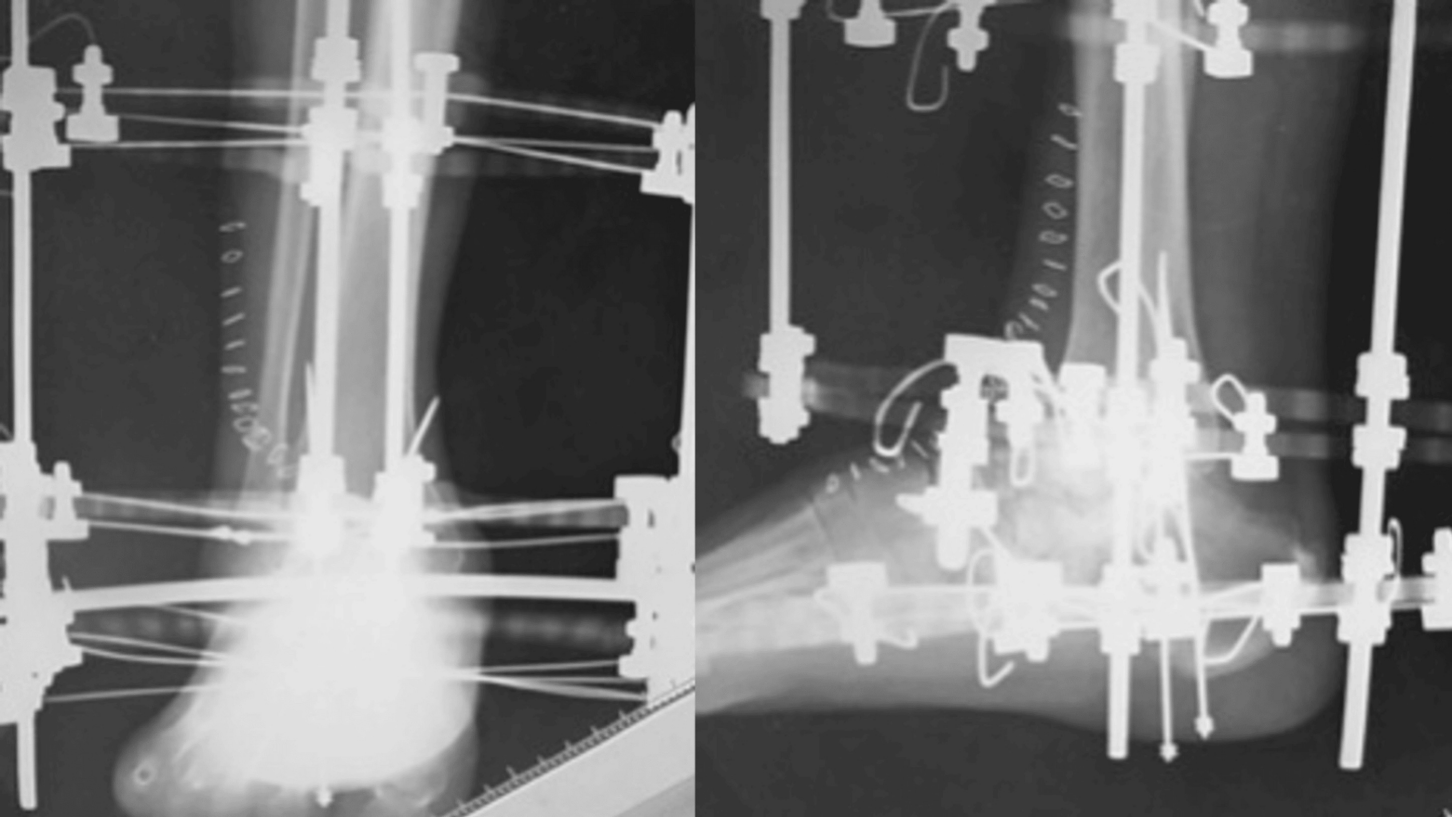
Postoperative radiographs
